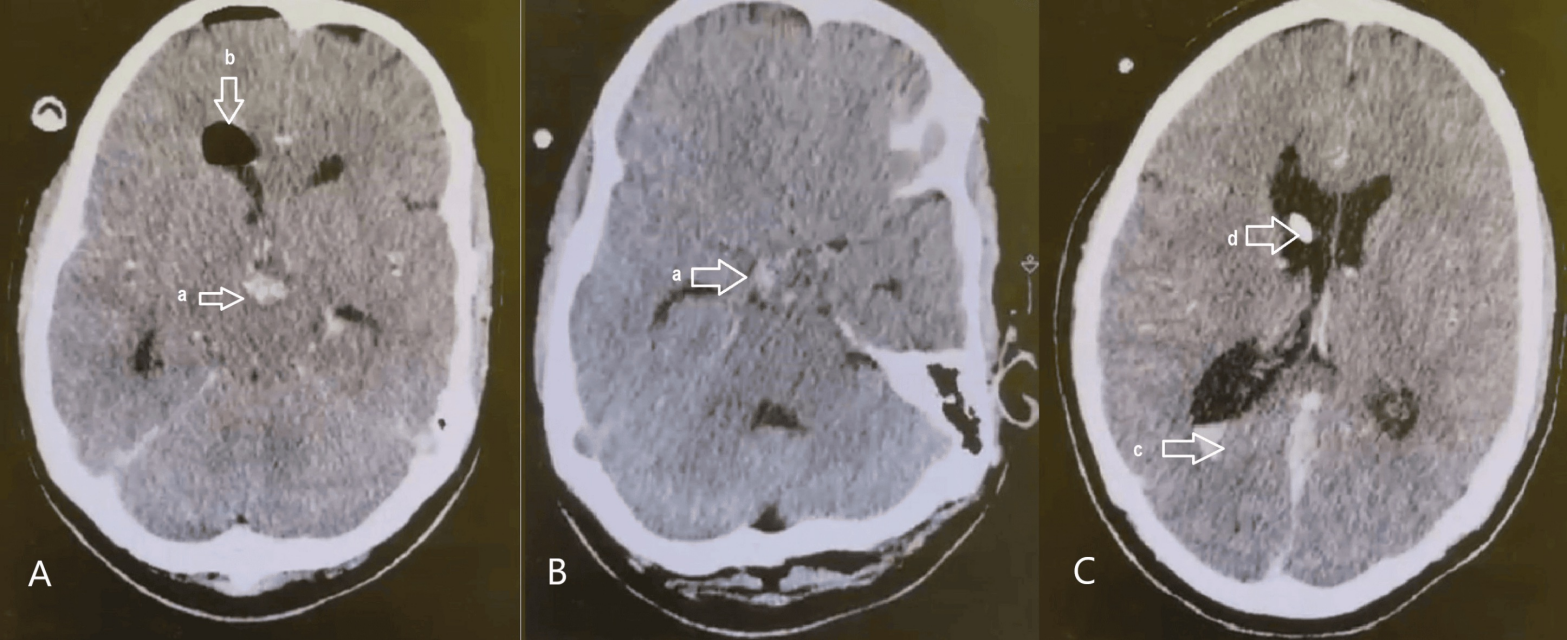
Follow-up brain CT of clinical case 2 scan showing residual tumor with signs of bleeding (a), pneumoventriculism (b), and intraventricular hemorrhage (c). A: Postoperative axial brain CT scan of clinical case 2 showing pneumoventriculism (b) and residual tumor (a) with hemorrhagic changes. B: Postoperative axial brain CT scan of clinical case 2 residual (a) tumor with hemorrhagic changes. C: Postoperative axial brain CT scan of clinical case 2 showing intraventricular hemorrhage (c) with external ventricular drainage catheter (d). a: residual tumor with signs of bleeding. b: pneumoventriculism. c: intraventricular hemorrhage. d: external ventricular drainage catheter.
